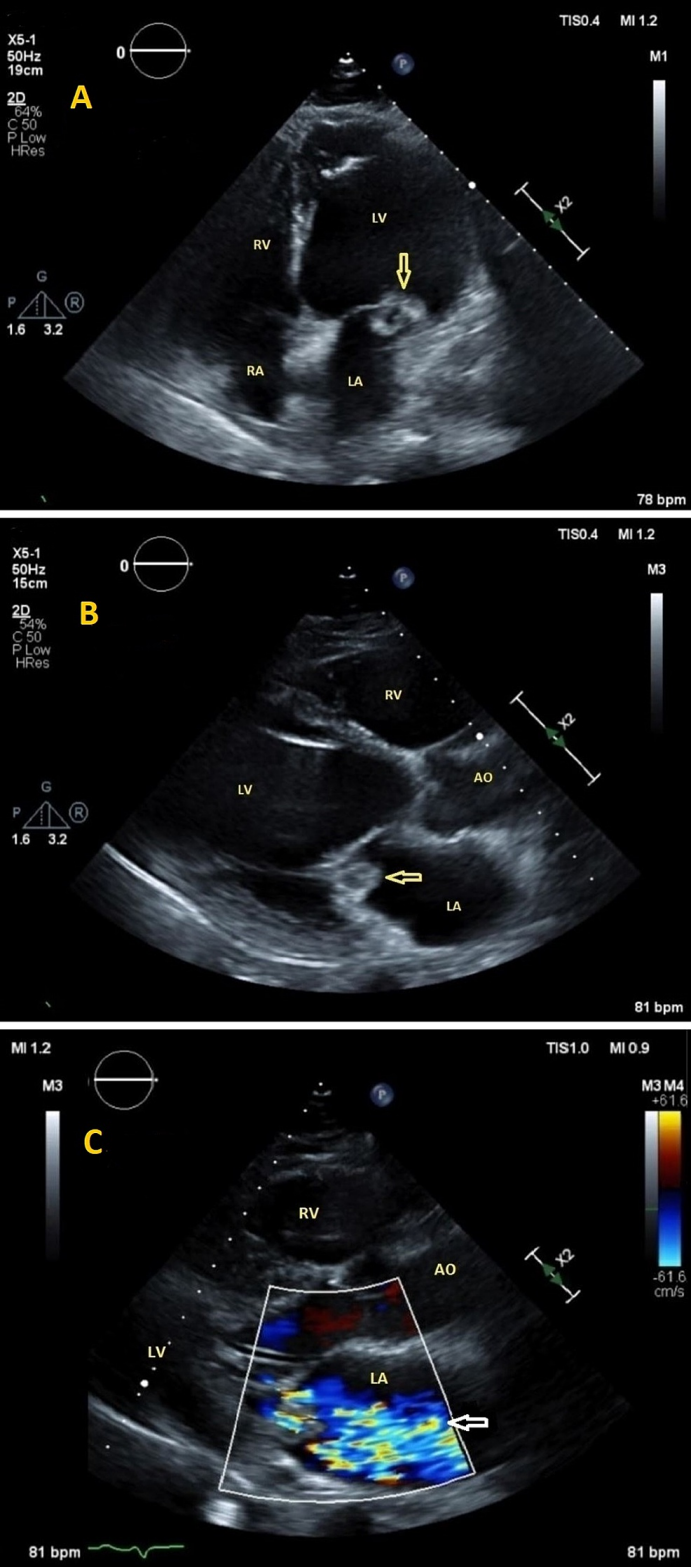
Transthoracic echocardiography 3A (apical 4 chamber view) and 3B (parasternal long-axis view) show a rounded irregular mass with a lucent center attached to the posterior leaflet of the mitral valve consistent with vegetation (yellow arrows), and a severely dilated left ventricle. 3C: Color Doppler showing severe mitral regurgitation (white arrow).
